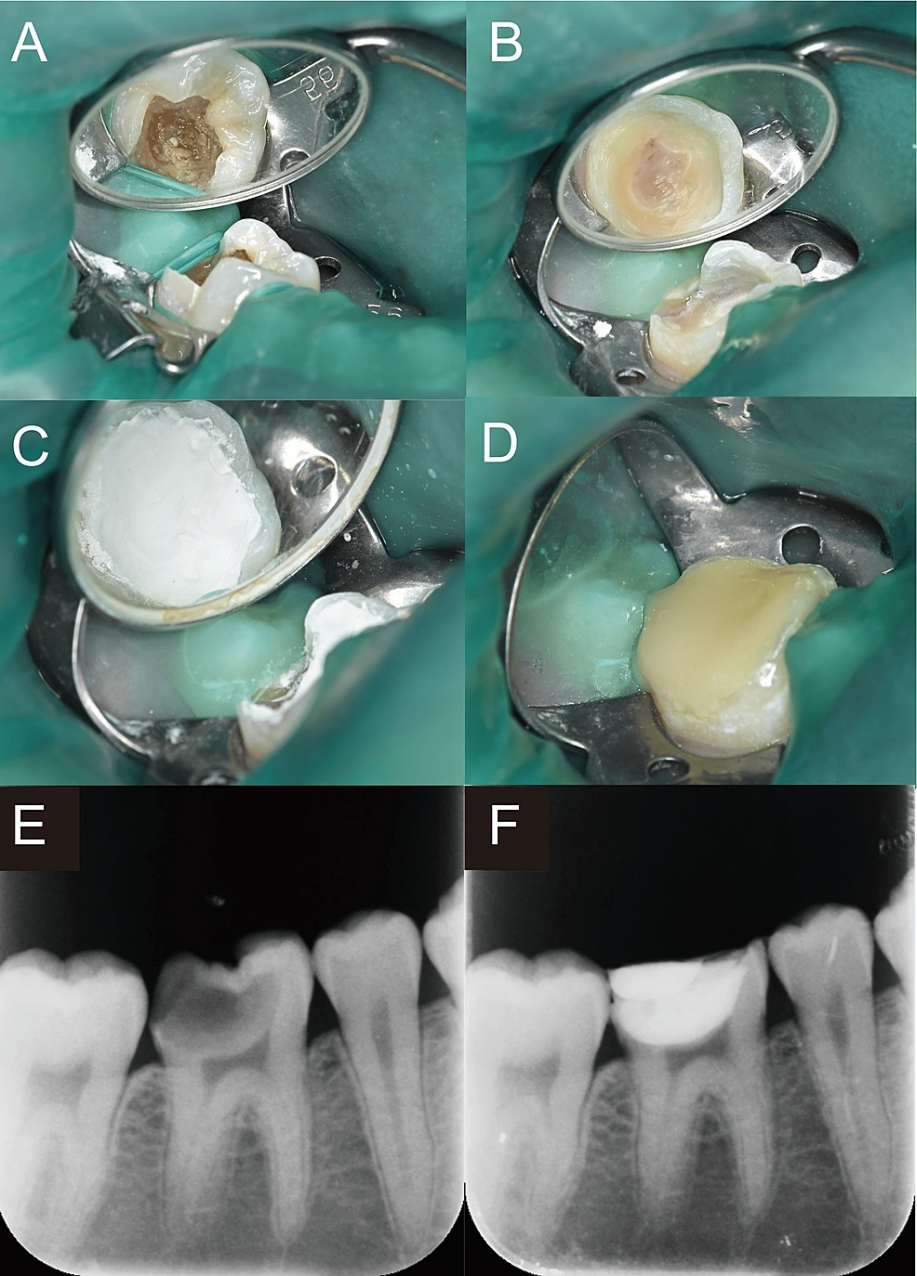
Clinical images and intraoral periapical radiographs of Case two. (A) Before treatment. (B) After removal of dental caries. (C) After placement of mineral trioxide aggregate cement over the dental pulp. (D) Preoperative radiograph. (E) Radiograph taken three months postoperatively.
